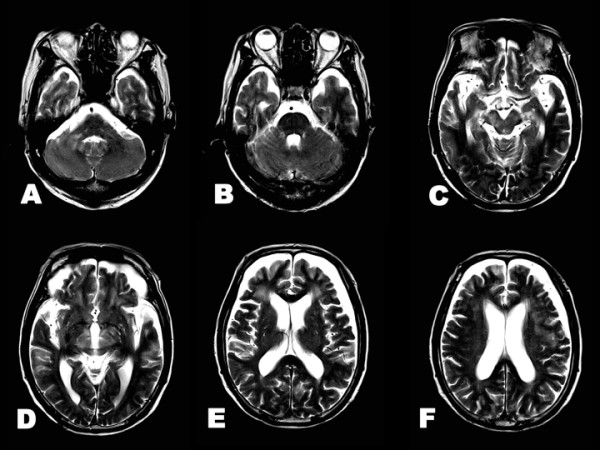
Brain T2-weighted imaging after neurological recovery. A-F. T2-hyperintense lesions are markedly attenuated. Hyperintense lesions remain in the right middle cerebellar peduncle, midbrain and cerebral white matter.
